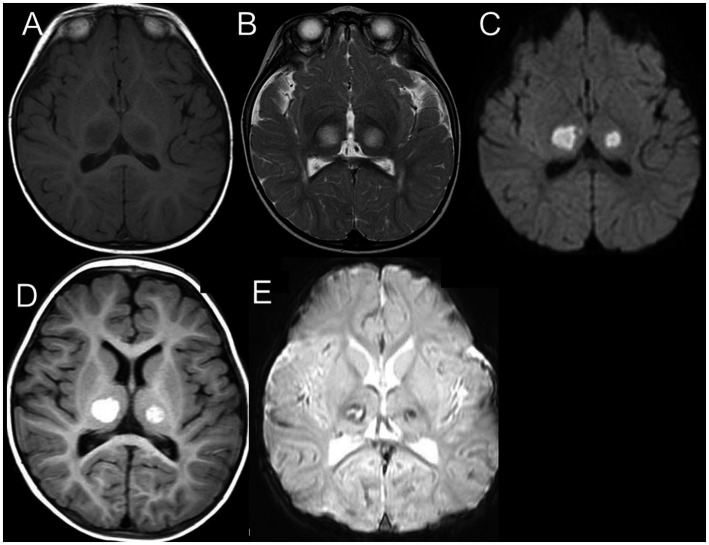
MRI of 1-year-old boy with ANE. MRI on day 4 reveals bilateral symmetric thalamic lesions [low on T1WI (A) and high on T2WI (B)] with reduced diffusion (C). MRI on day 14 shows high intensity on T1WI (D) with partly low intensity on T2 star-weighted imaging (E), suggesting hemorrhagic degeneration (from Takanashi, 2018 with permission). MRI, magnetic resonance imaging; ANE, acute necrotizing encephalopathy; T1WI, T1-weighted imaging; T2WI, T2-weighted imaging.
